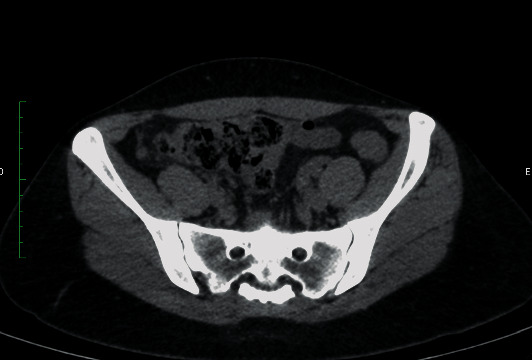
Computed tomography—axial section.
